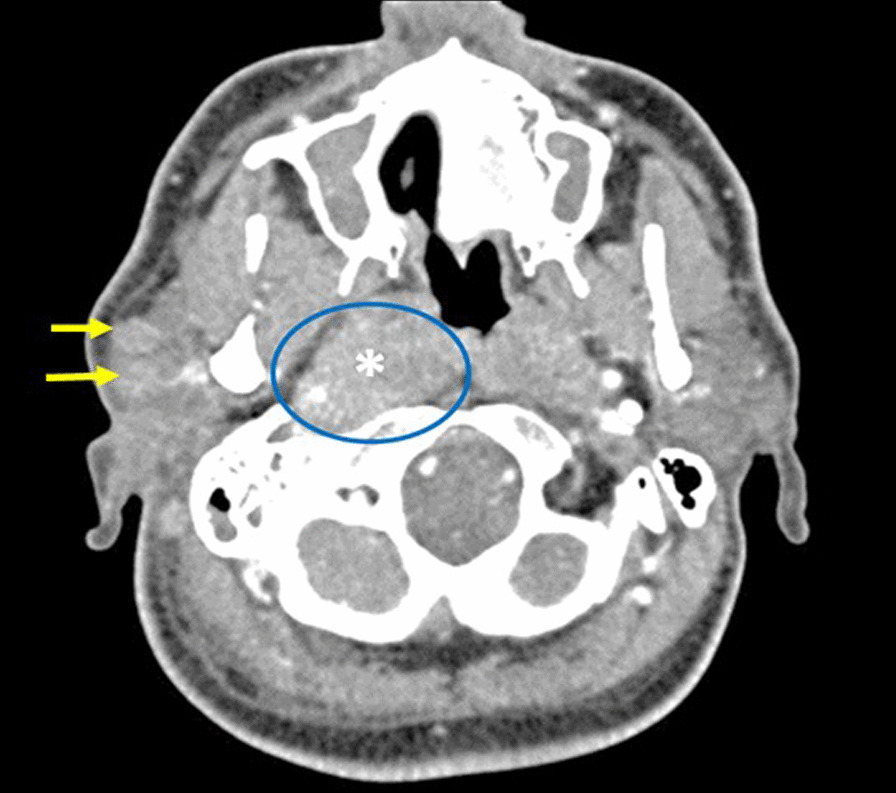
Venous phase cervical CT (soft tissue window axial cut) shows nasopharyngeal carcinoma centered in right Rosenmüller fossa (asterisk) with deep posterior extension into carotid space and heterogeneous masses (arrows) of the right parotid
